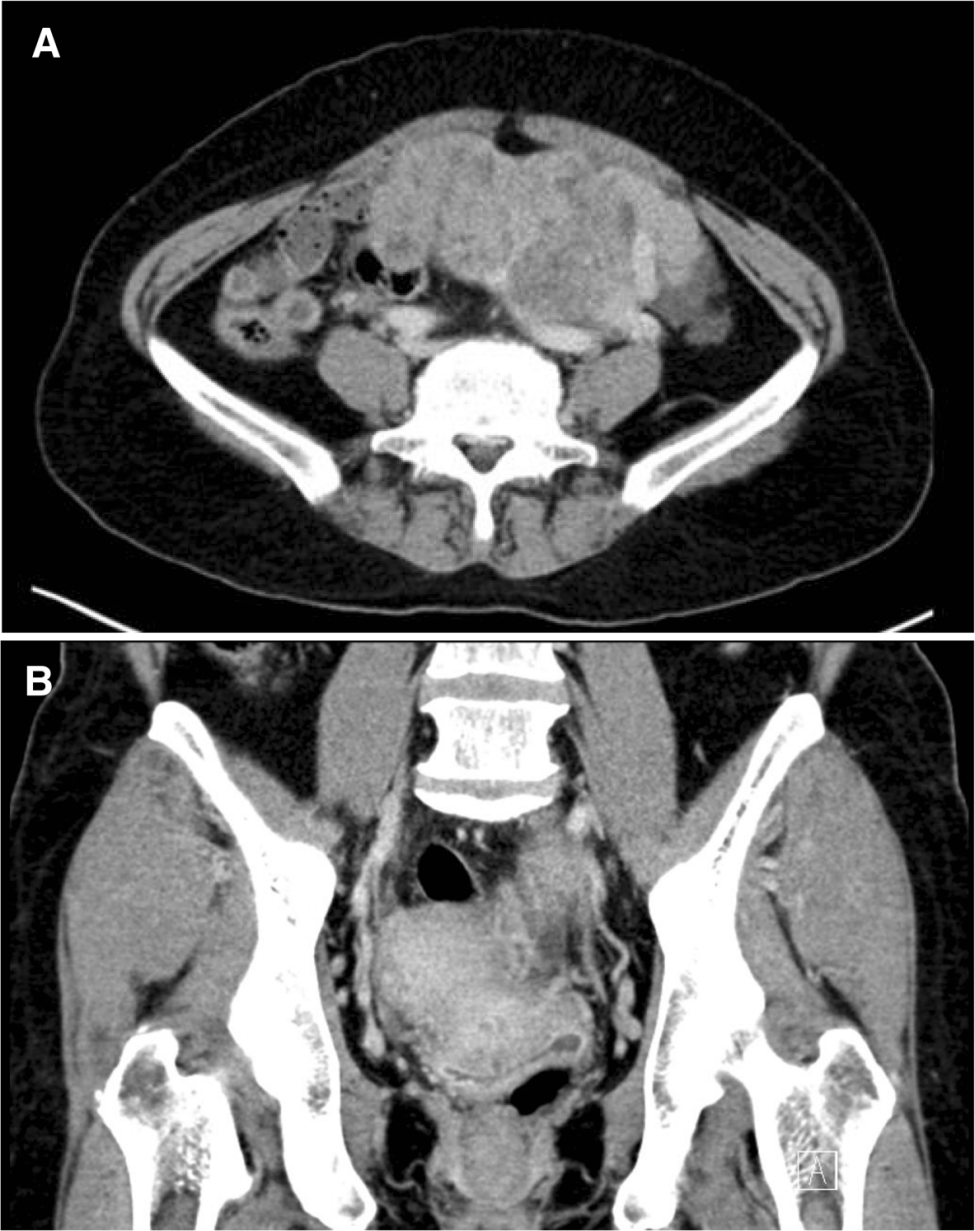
Computed tomography (CT) images showed lobulated soft tissue density mass (9.0 × 13.6 cm) suggesting left ovarian cancer. (a) axial image. (b) coronal image
